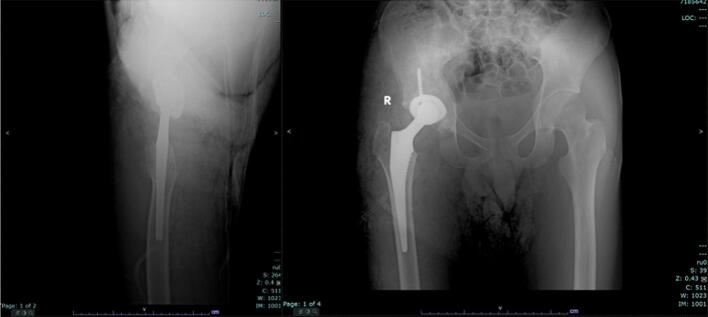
Post-operative plain radiograph of pelvic AP/Lat view.
